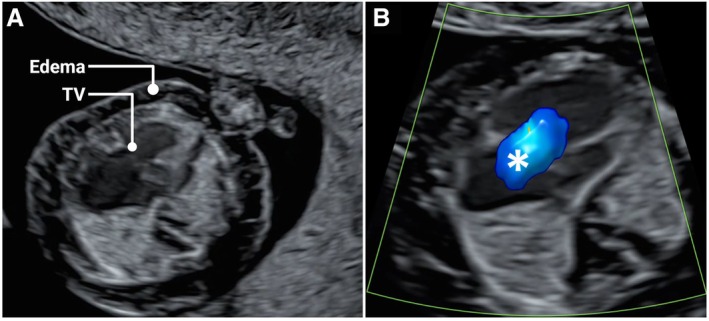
Ebstein anomaly was diagnosed at 12^+5^ weeks in this fetus with marked edema and tricuspid valve insufficiency (A). Color Doppler showed tricuspid regurgitation during systole (asterisk) (B) (TV, tricuspid valve).
